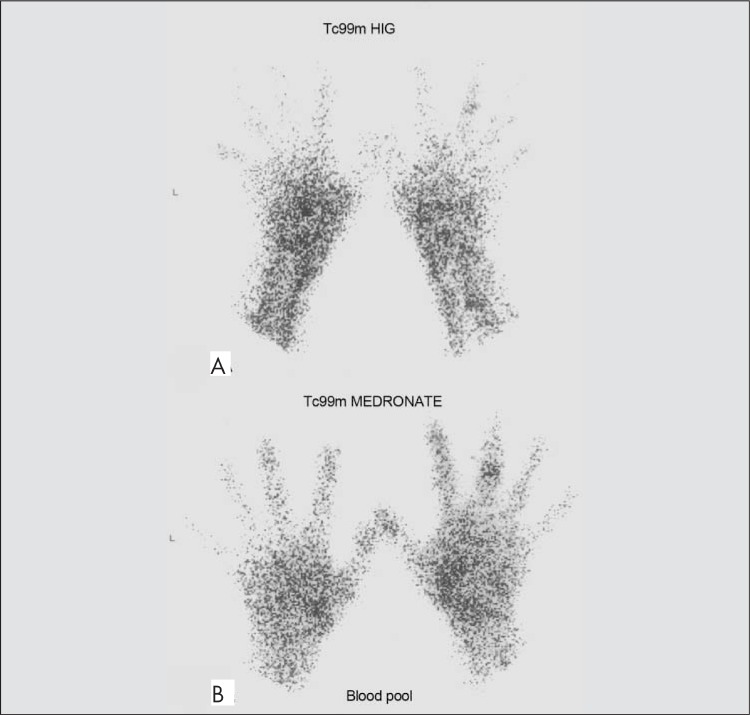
Tc 99m HIG scintigraphy (A) and Tc 99m Medronate bloodpool scintigraphy (B) in the same patientFaint uptake (VAJS= 1) in the proximal interphalangeal joint (at 3^rd^ phalanx of theright hand) and normal uptake of radiopharmaceutical at the level of backgroundactivity (VAJS=0) for other joints of both hands and wrists in HIG and Medronateblood pool scintigraphy
